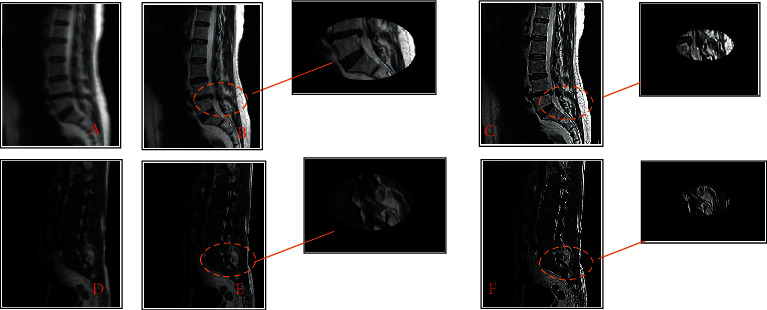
Comparison of MRI images of patients with spinal metastatic tumors processed by different algorithms.
